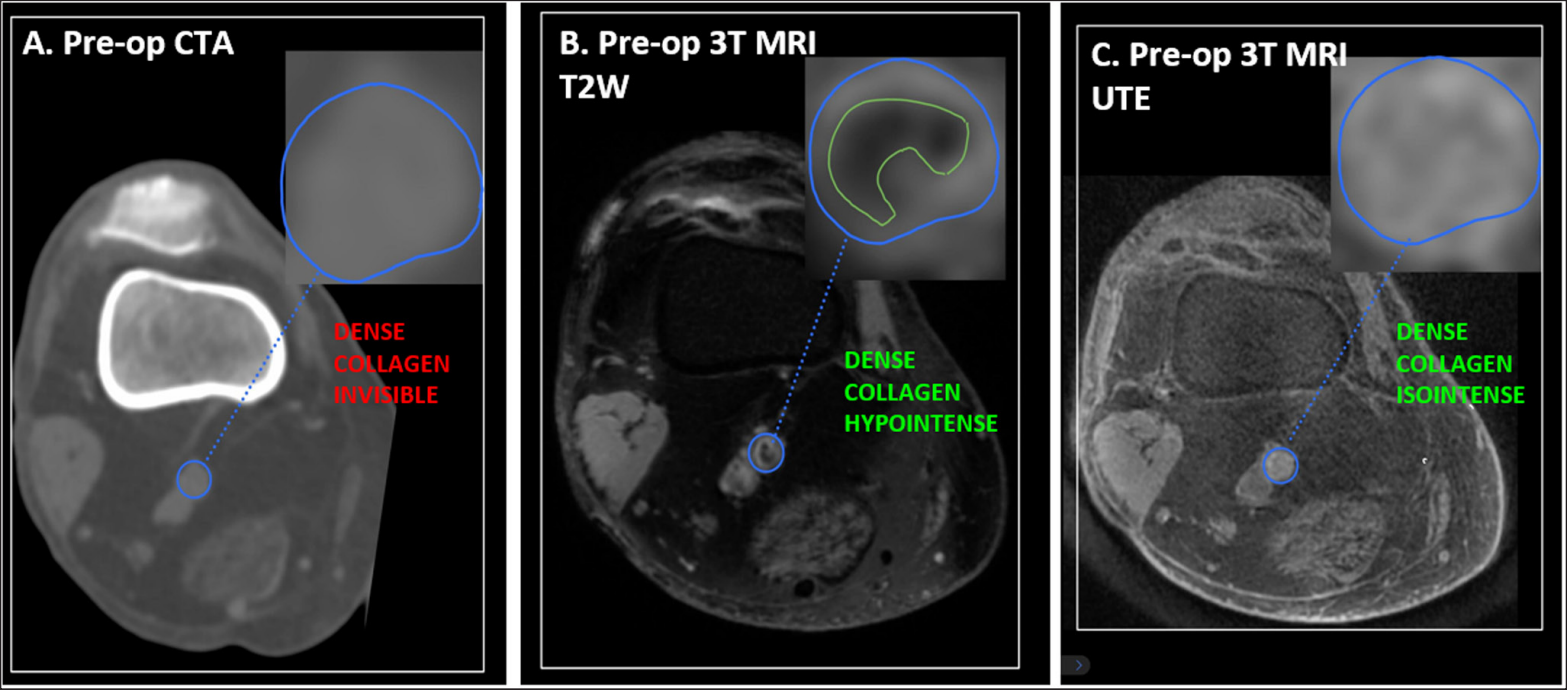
Preoperative (pre-op) imaging with computerized tomographic angiography (CTA) and magnetic resonance imaging (MRI). CTA shows an occluded popliteal artery isodense to the reference muscle tissues (A). MRI shows a lesion that is hypointense in T2-weighted (B)and isointense in ultrashort echo time (C), characteristic of dense collagen.
